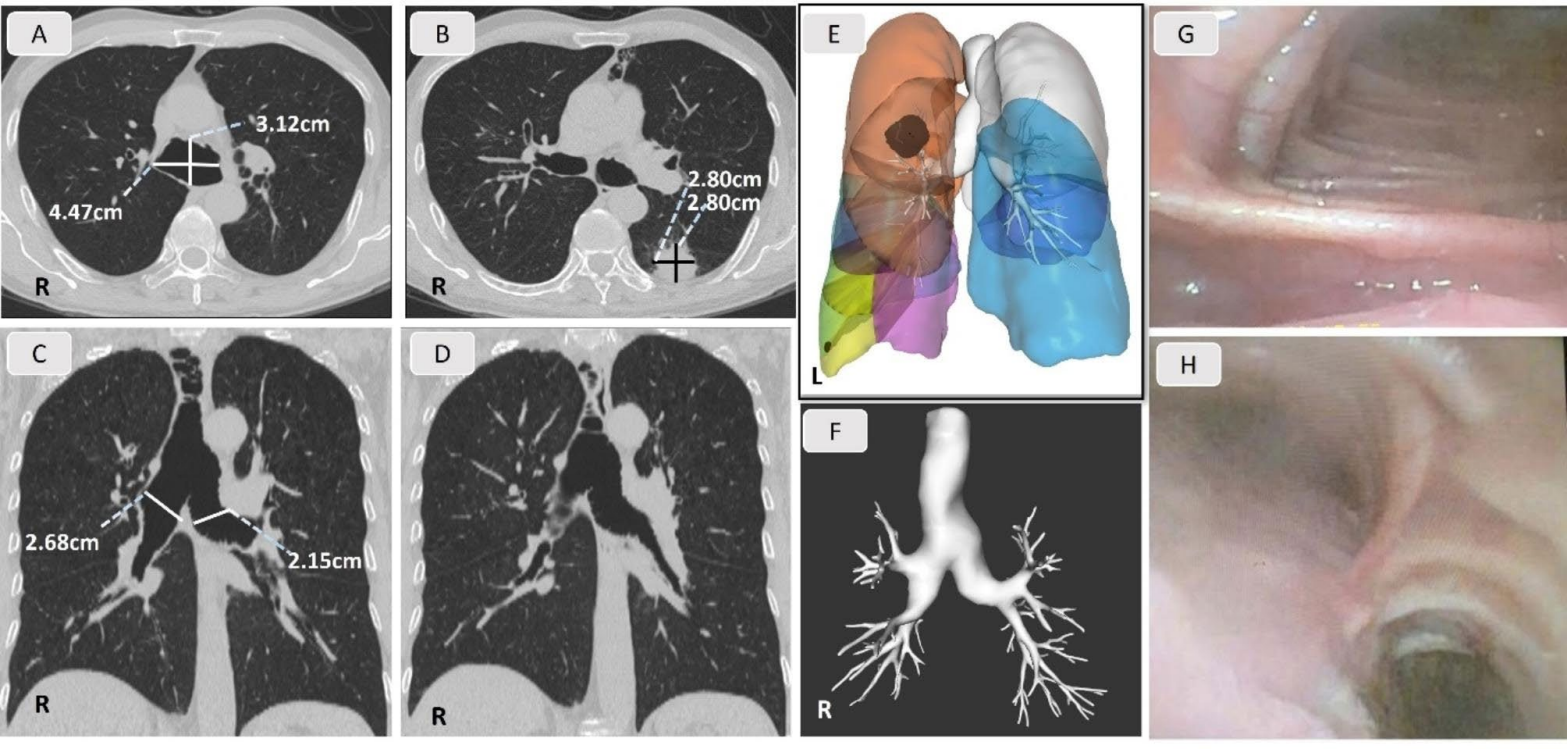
Preoperative imaging and fiberoscopic findings. a-b. The transverse thoracic CT. The maximum anteroposterior and axis diameters of the trachea and the tumor. c-d. The coronal thoracic CT. The maximum diameters of the left and right main bronchus and the deformed central airway with multiple diverticula. e-f. Coronal thoracic CT reconstruction. The tumor and the distorted airway. g-h. Fiberoptic bronchoscopy. The trachea and the major bronchi at the carina
